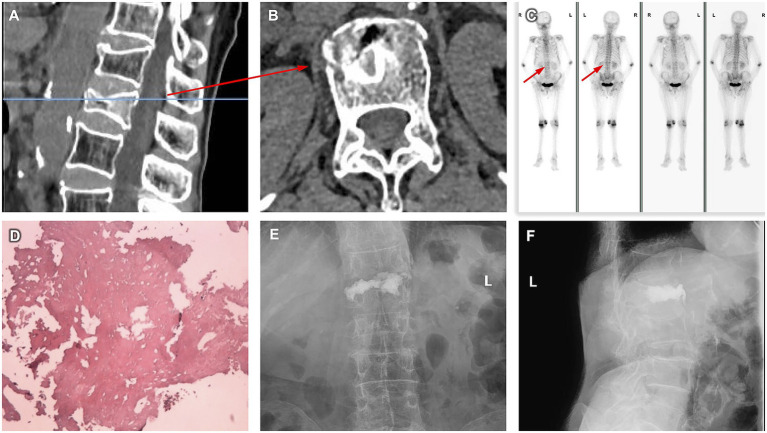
Multimodal imaging and clinical-pathological features of KD in the reparative phase (Case 1). (A,B) Preoperative plain radiographs. The anteroposterior and lateral views reveal generalized osteopenia and a significant anterior wedge compression deformity of the L1 vertebra, accompanied by focal kyphotic angulation. (C,D) Preoperative sagittal and axial CT scans. A distinct, horizontally oriented intravertebral vacuum cleft (IVC) is clearly visible within the collapsed L1 vertebral body. This radiolucent shadow represents the accumulation of nitrogen gas within the necrotic cavity. The surrounding cancellous bone exhibits reactive osteosclerosis and trabecular impaction, without significant retropulsion of the posterior vertebral wall into the spinal canal. (E)
